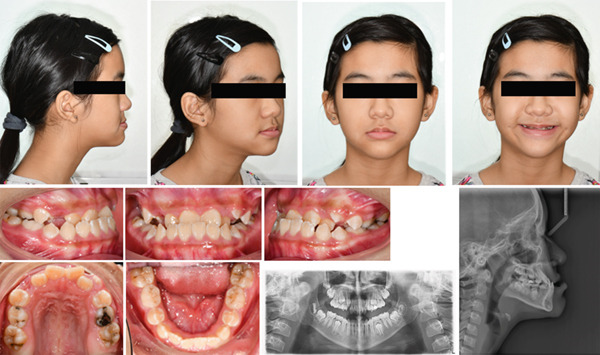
Pretreatment extraoral and intraoral photographs, panoramic radiograph and lateral cephalometric radiograph demonstrating a skeletal Class III pattern with anterior crossbite and reduced incisal display.
